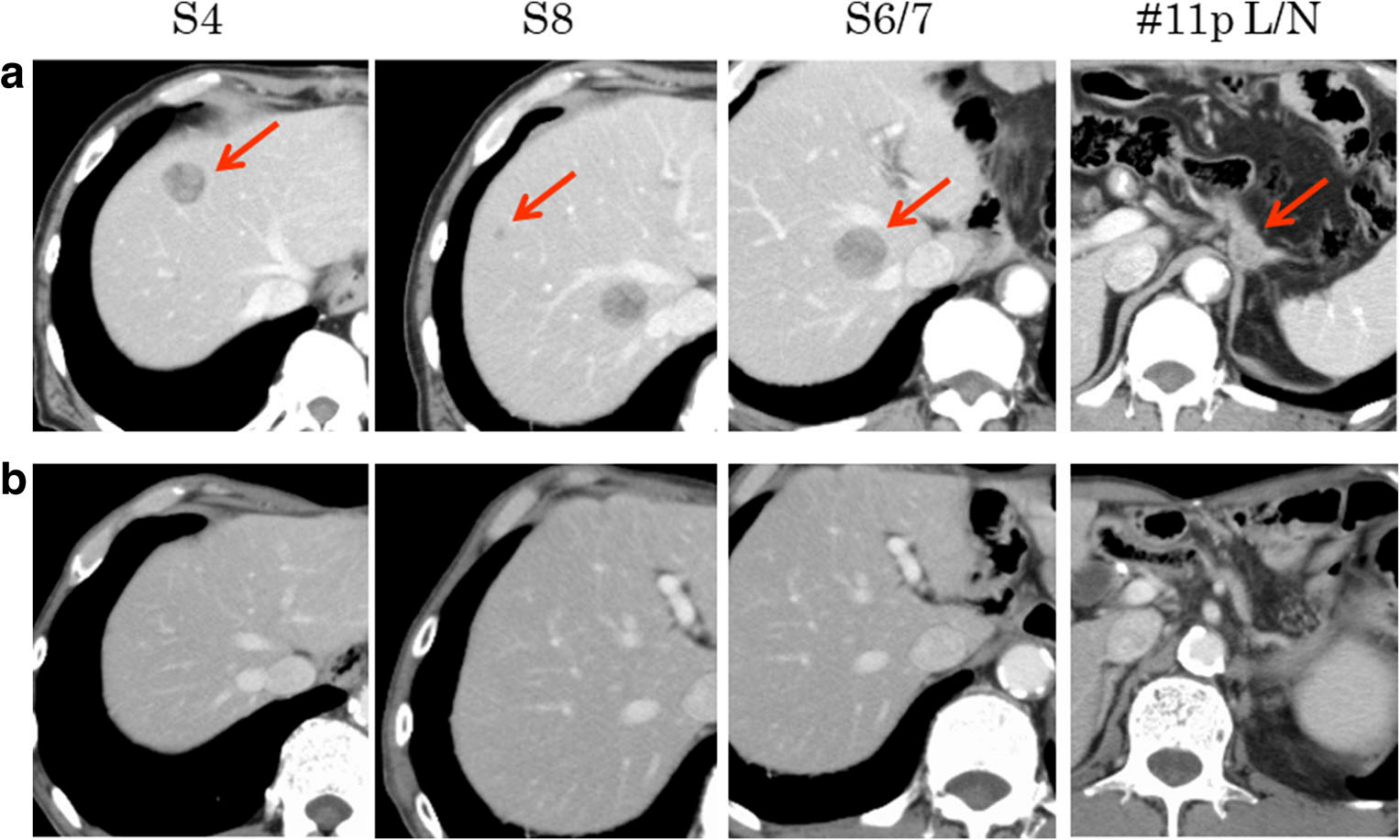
The changes in the CT findings during multidisciplinary therapy. a CT showed multiple metastases in the lymph nodes and liver. b After chemotherapy and percutaneous ethanol therapy (PEIT), all of the metastatic lesions disappeared
